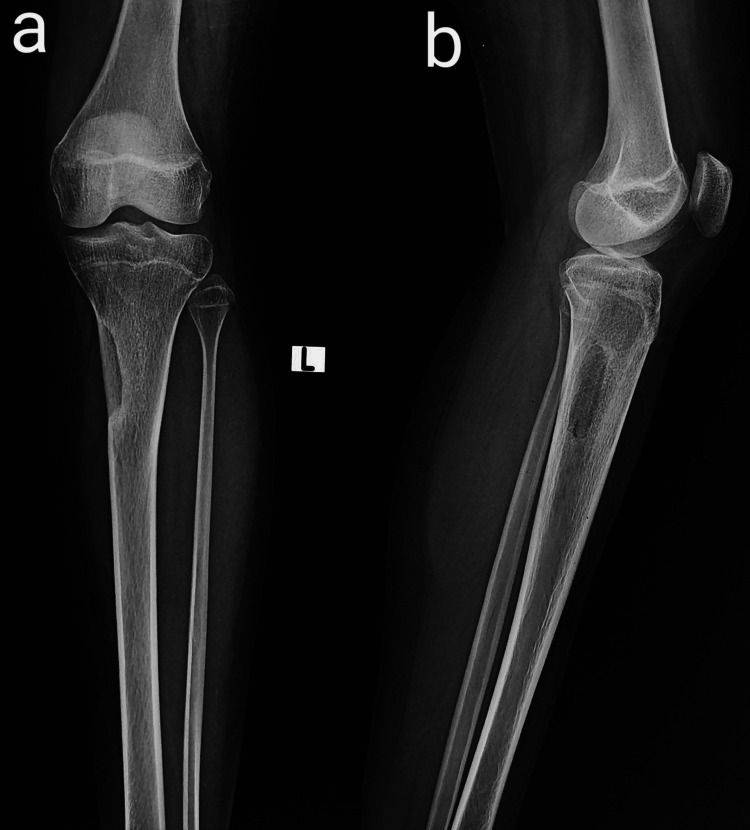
Anteroposterior (a) and lateral (b) plain radiograph of the left knee with the leg (six month postoperative follow-up) No evidence of recurrence
